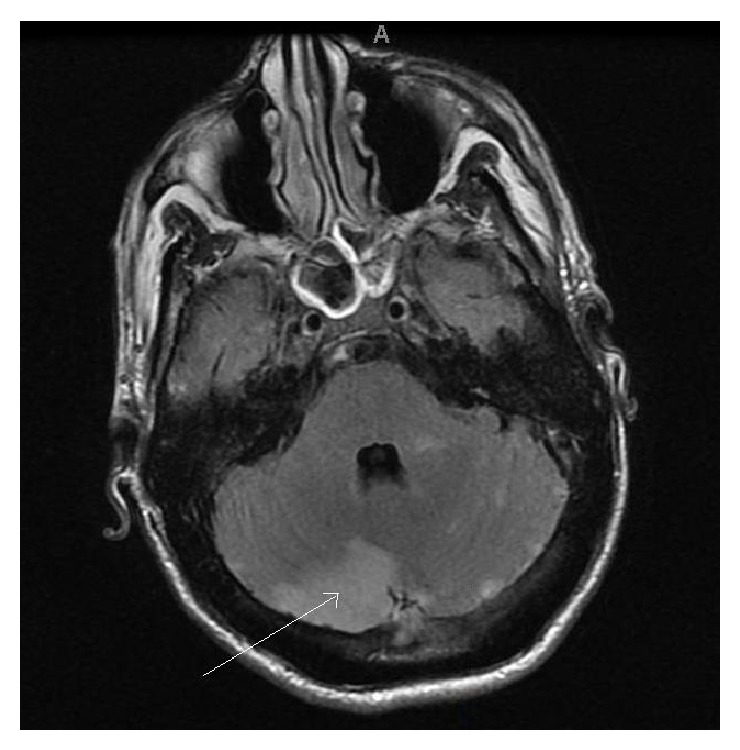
MRI brain with and without contrast revealing multiple foci of predominantly cortical signal change with the largest area involving the medial right cerebellar hemisphere (white arrow). The appearance is most compatible with subacute infarcts, presumably from an embolic source. There are no enhancing masses to suggest metastatic disease.
